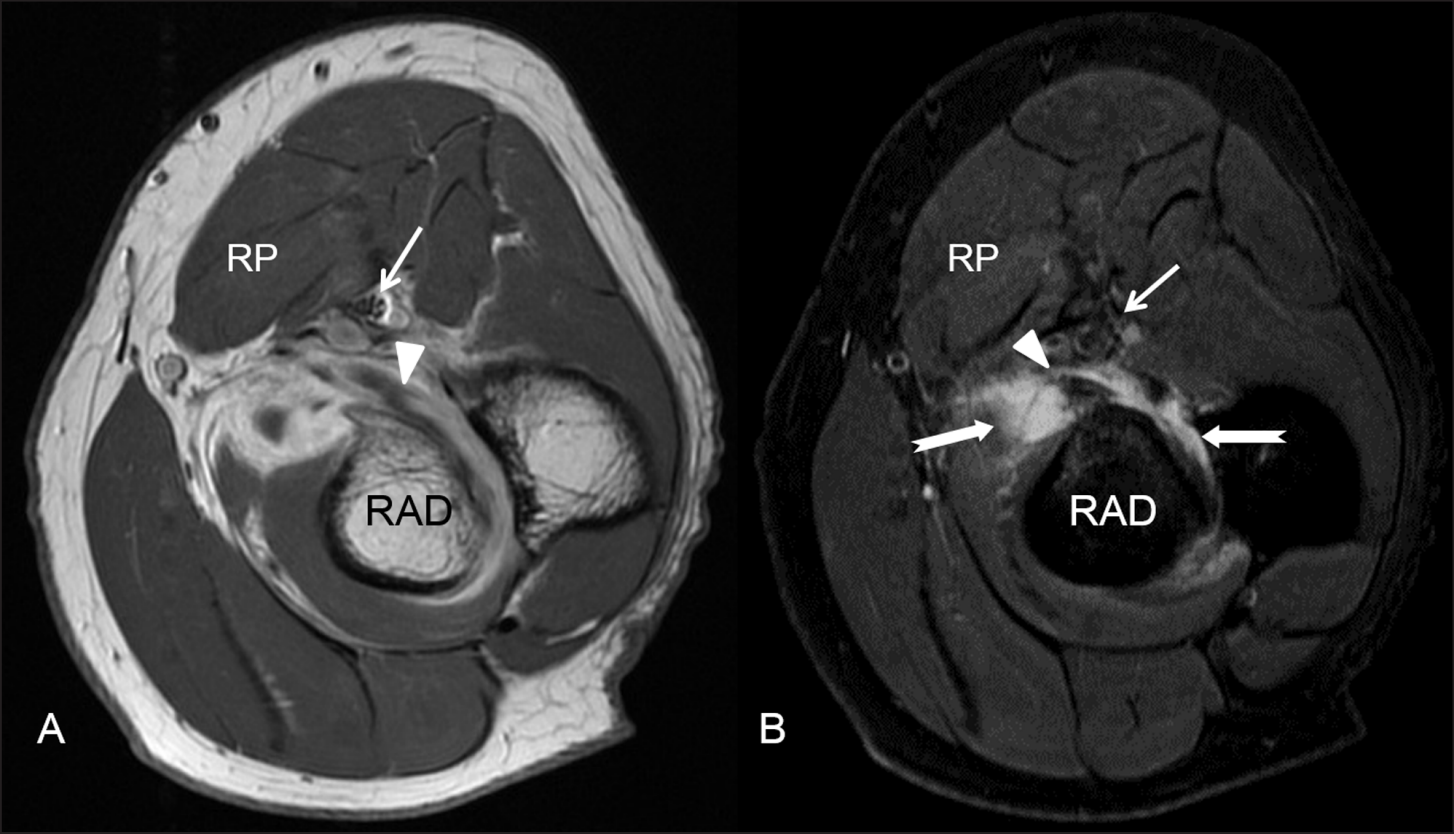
Median nerve entrapment neuropathy caused by an extrinsic compression due to a bicipital bursa. MRI with axial views: T1-weighted A) and T2-Weighted Fat Sat sequences B). RP = pronator teres muscle, RAD = radius. The median nerve (small white arrow) is located close to the bursa (white arrows) surrounding the insertion of the distal biceps tendon on the radial tuberosity.
